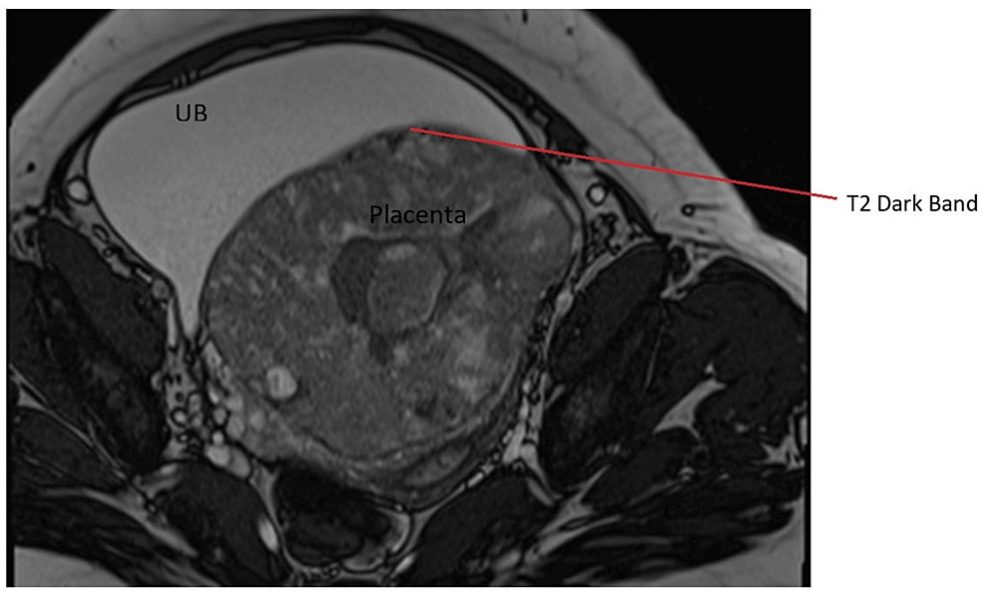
MRI scan image (axial view) Axial view showing a T2 dark band and placental bulging into the posterior wall of the urinary bladder (UB) with a loss of placental-myometrial junction MRI: magnetic resonance imaging
